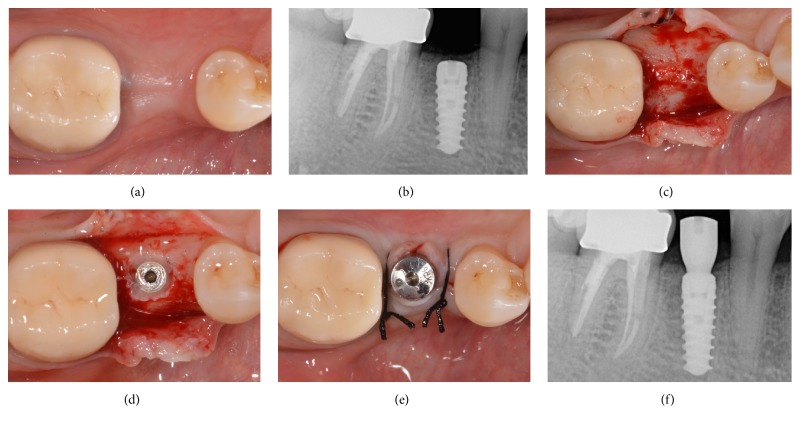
Immediate implant placement of a mandibular second premolar (#45). Soft tissue healing after 3 months (a); periapical radiograph after 3 months of submerged healing (b); second-stage surgery and the alveolar ridge 3 months after implant placement (c); fixture exposure during second-stage surgery (d); placement of the healing abutment and sutures (e); periapical radiograph after insertion of the healing abutment (f).
